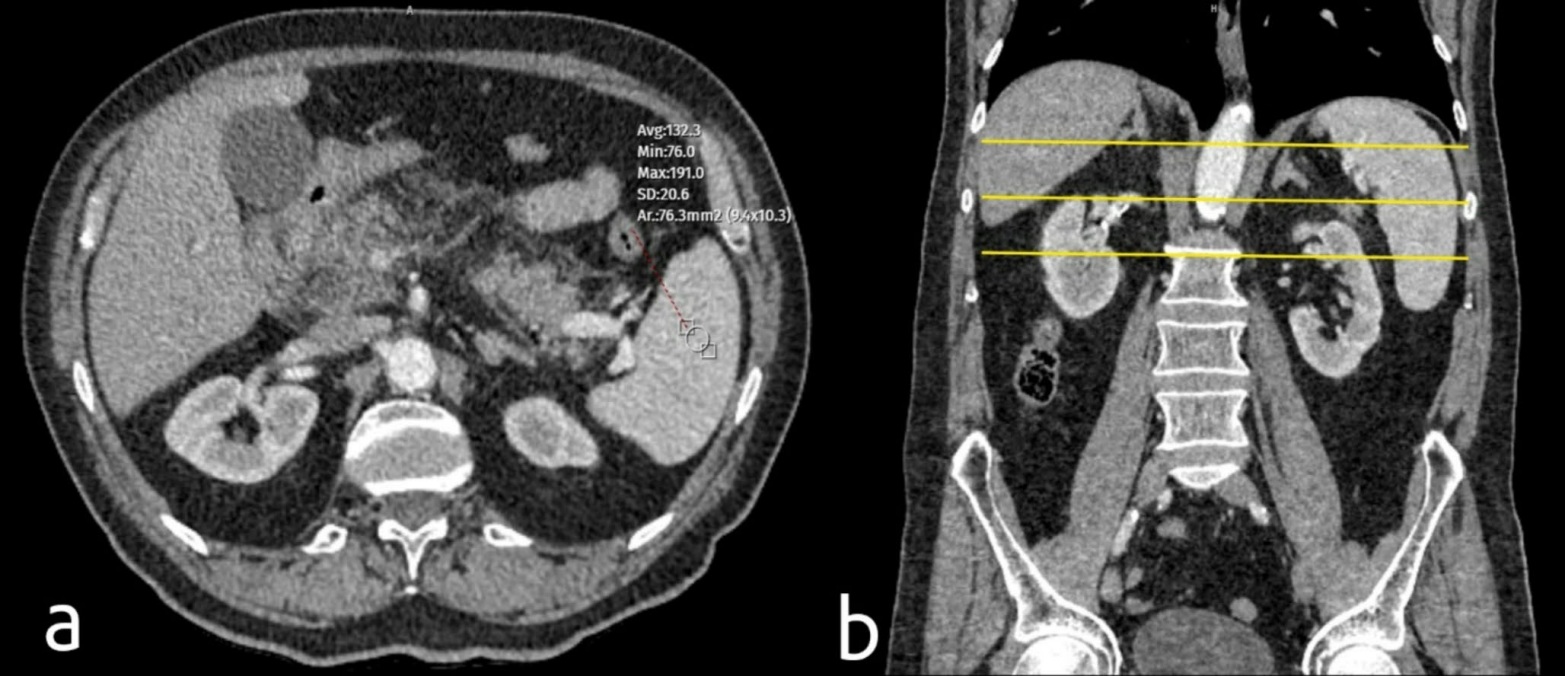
(a) Axial contrast-enhanced CT image showing a measurement of splenic attenuation with an average value of 132.3 HU. (b) Coronal contrast-enhanced CT image demonstrating the three axial levels (marked with yellow lines) where splenic attenuation measurements were performed. The mean splenic attenuation value was calculated using the average of these three measurements
